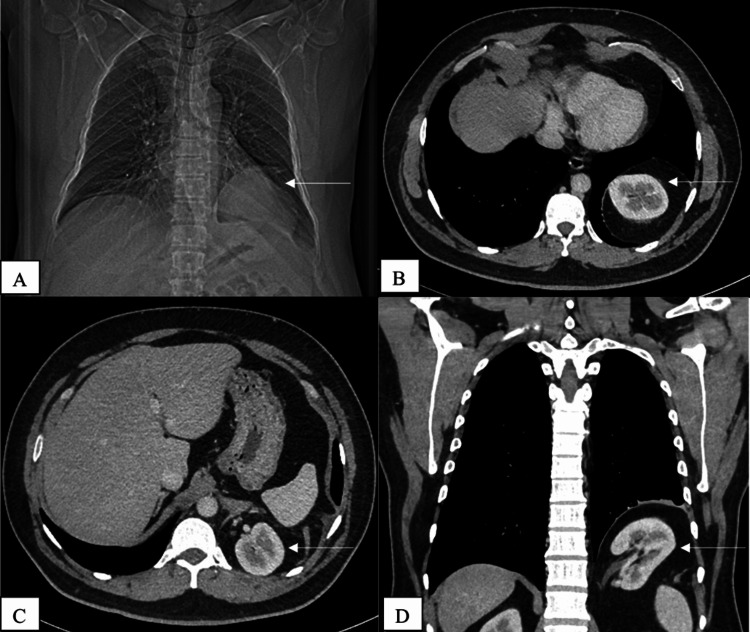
Bochdalek hernia with intra-thoracic kidney (A) Chest X-ray showing a well-defined radio-opaque lesion in the lower left zone (white arrow). (B) Axial view of contrast-enhanced computed tomography (CT) chest showing left intra-thoracic ectopic kidney (white arrow). Axial (C) and coronal (D) views of contrast-enhanced CT chest and abdomen showing an intra-thoracic kidney and Bochdalek hernia (white arrow).
